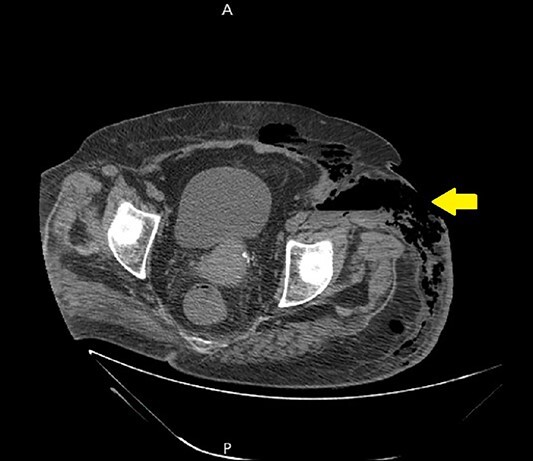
A non-contrast abdomen and pelvis CT scan, which shows a skin defect with underlying abscess collection and air–fluid level, with significant subcutaneous air collection.
